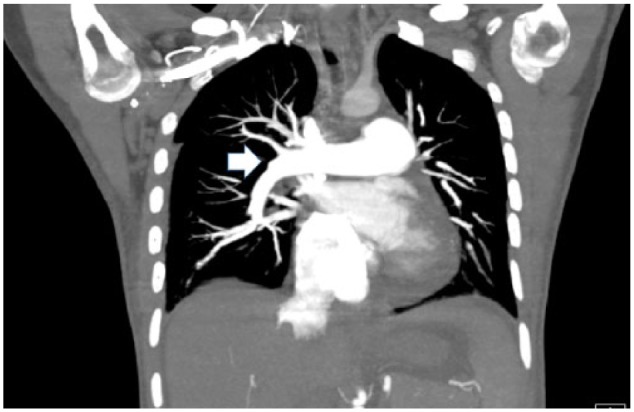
Computed tomography with contrast, coronal cut showing large pulmonary arterial trunk (arrow).
